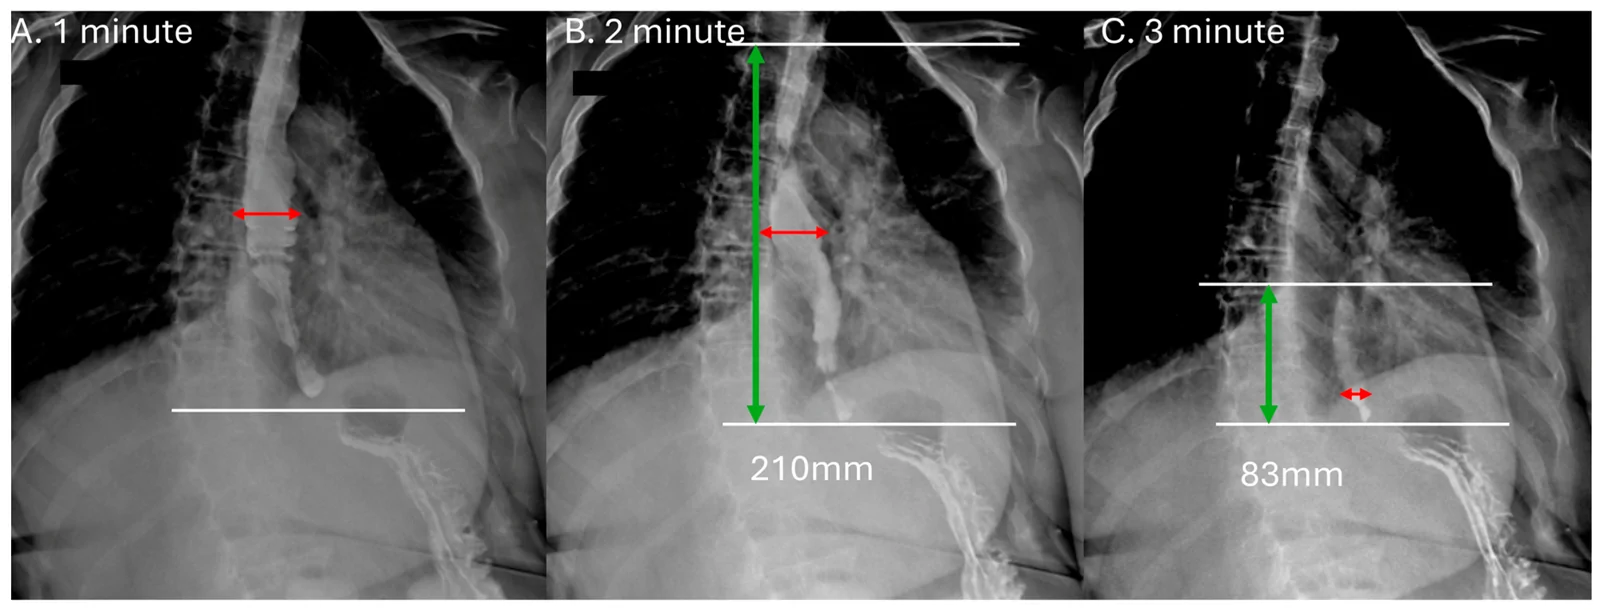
Representative timed barium esophagogram showing progressive reduction in retained barium. Left posterior oblique radiographs obtained at 1 min (A), 2 min (B), and 5 min (C) after barium ingestion demonstrate persistent but progressively decreasing esophageal barium retention. The vertical green line indicates barium column height, measured between the upper and lower limits of the retained contrast column, while the horizontal red arrow indicates maximal column width. In this example, barium column height decreased from 210 mm at 2 min to 83 mm at 5 min, indicating partial but incomplete clearance.
